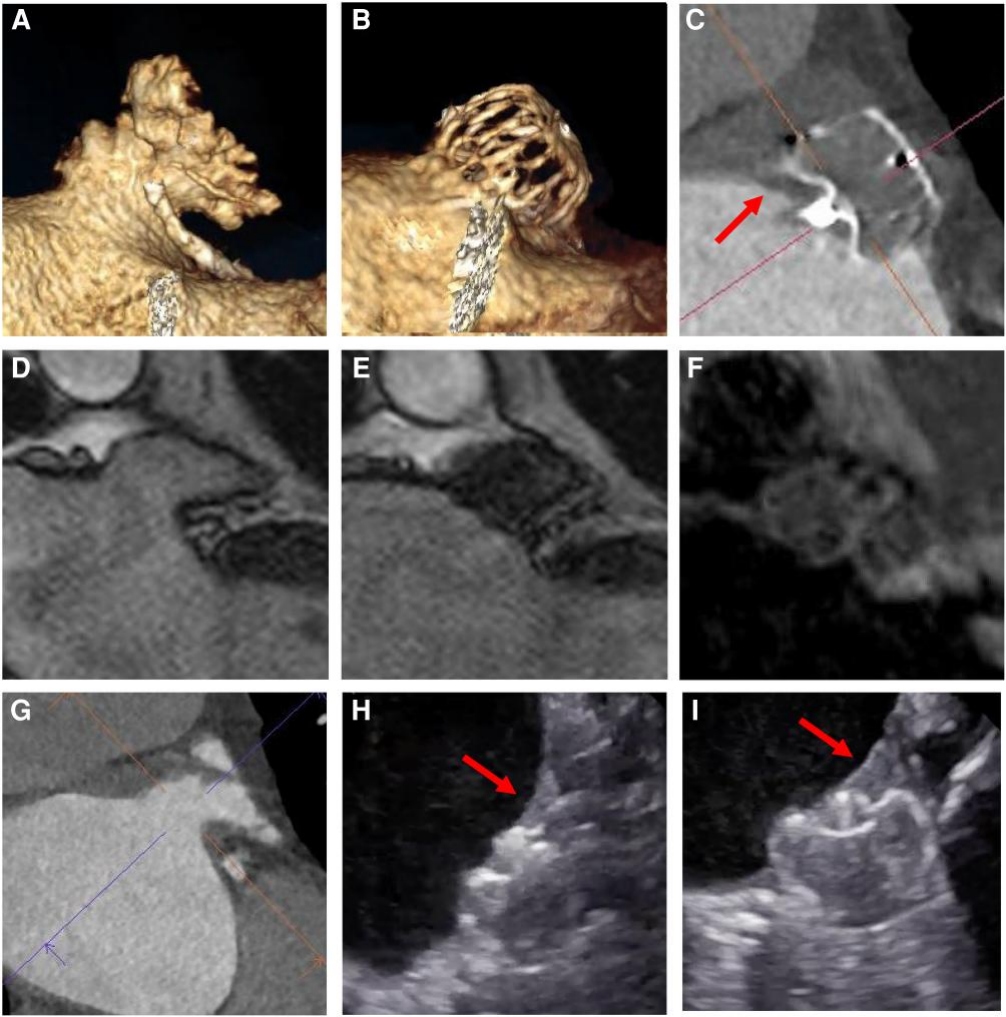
Cardiac computed tomography, transoesophageal echocardiography, and magnetic resonance imaging images obtained before left atrial appendage closure and at Day 45 after implantation of the first-in-human WATCHMAN FLX Pro device. (*A* and *G*) Computed tomography images of the left atrial appendage before closure showing a chicken wing anatomy. (*B* and *C*) Computed tomography images of the device implanted in the left atrial appendage showing complete closure. (*C*) Computed tomography showing hypoattenuated thickening at the upper half of the device with a smooth surface in continuation with the left atrial wall (arrow). (*D* and *E*) Magnetic resonance images showing the left atrial appendage before and after closure. (*F*) Specific bright-blood and black-blood phase sensitive magnetic resonance scan without any signs of fresh thrombus in the region of the hypoattenuated thickening. (*H* and *I*) Transoesophageal echocardiography images showing a layering on the upper half of the device with smooth surface and continuation with the left atrial wall (arrows).
